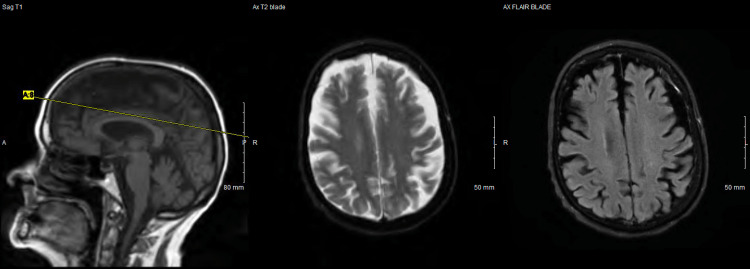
Magnetic resonance imaging with/without contrast of the head 2022 (3)
